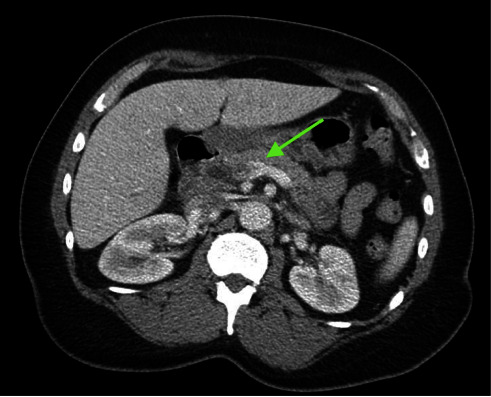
Axial section obtained from CT of the abdomen and pelvis with intravenous contrast demonstrates superior mesenteric vein thrombosis with surrounding inflammation consistent with thrombophlebitis (green arrow).
